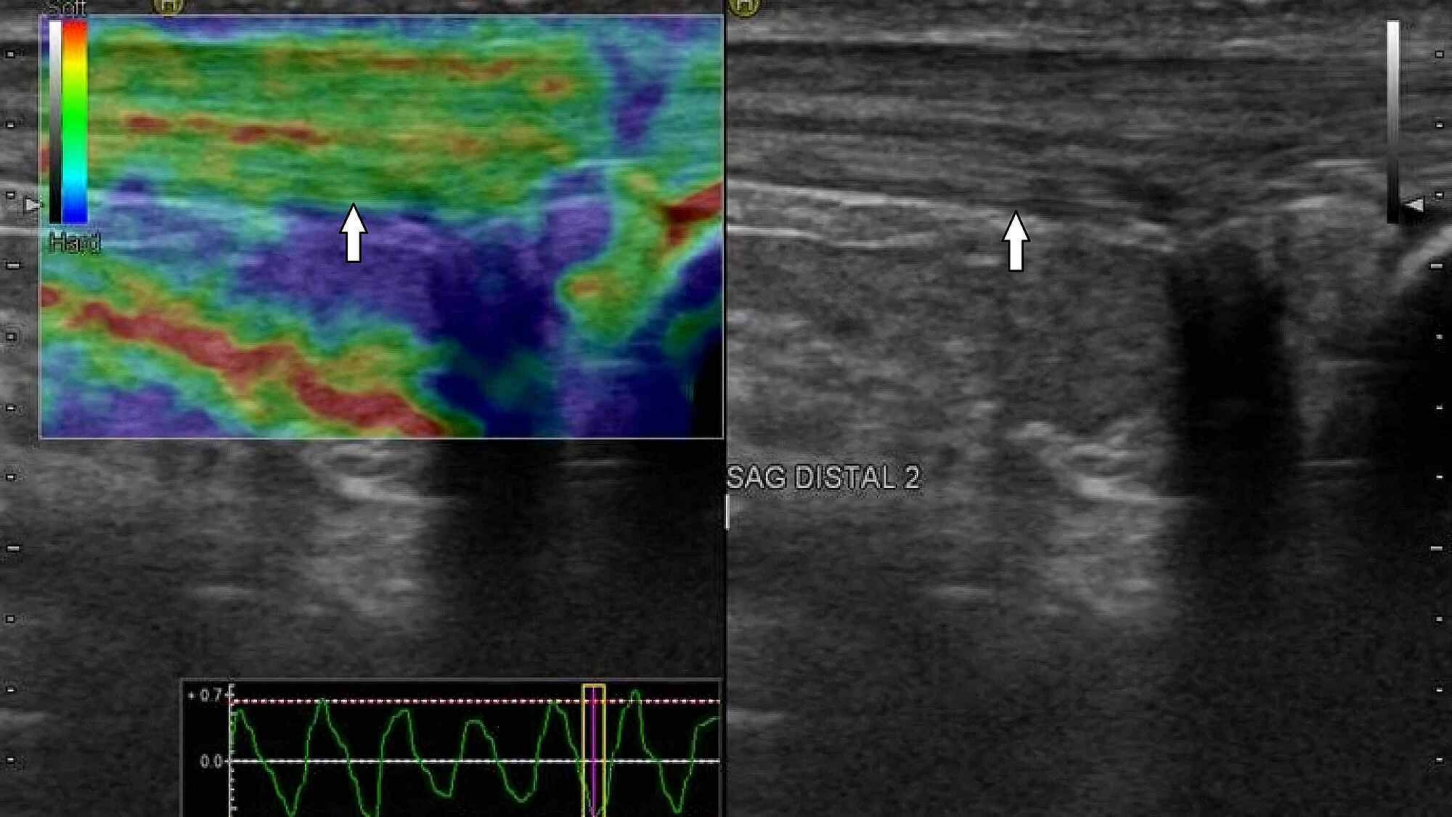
Grade 2 Achilles tendon. B-mode (right image) and sonoelastographic (left image) ultrasound images of the distal part of right Achilles tendon (arrows) in a 46-year-old healthy woman.
